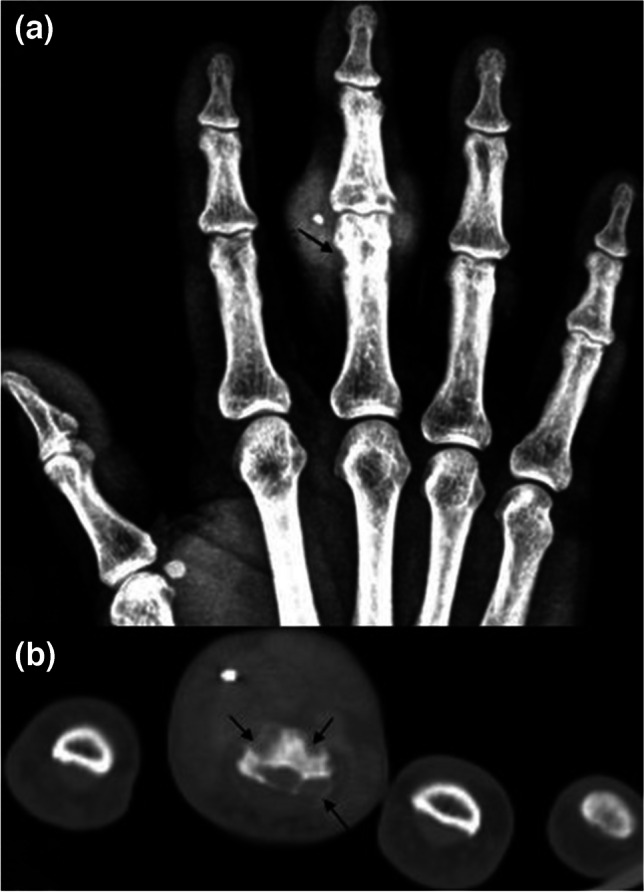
The 72-year-old man presented with prolonged pain for 2 years after hitting his hand on a solid object. A soft tissue mass-like swelling of proximal interphalangeal joint of the 3rd finger is noted. Anteroposterior radiograph (a) and axial bone window CT (b) images displayed erosions (arrows) on proximal phalanx along with a metallic foreign body impacted in the soft tissues. The large soft tissue swelling, representing foreign body granuloma, could mimic a tumor. MRI was not performed in this patient due to the potentially ferromagnetic properties of the foreign body within the soft tissue. A needle tip fragment was removed surgically
